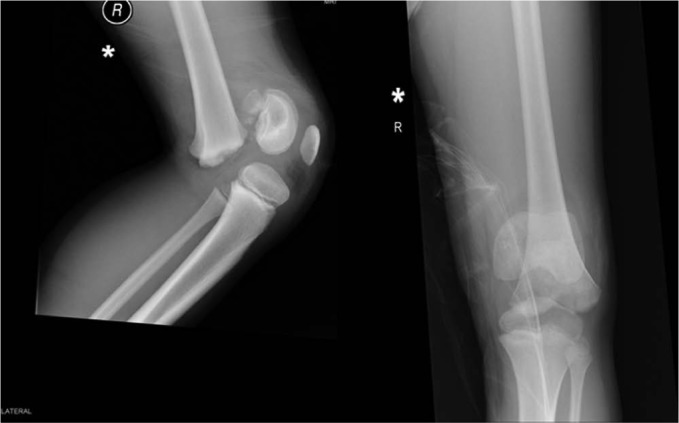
AP and lateral radiographs showing SH-2 injury of the distal femur.
